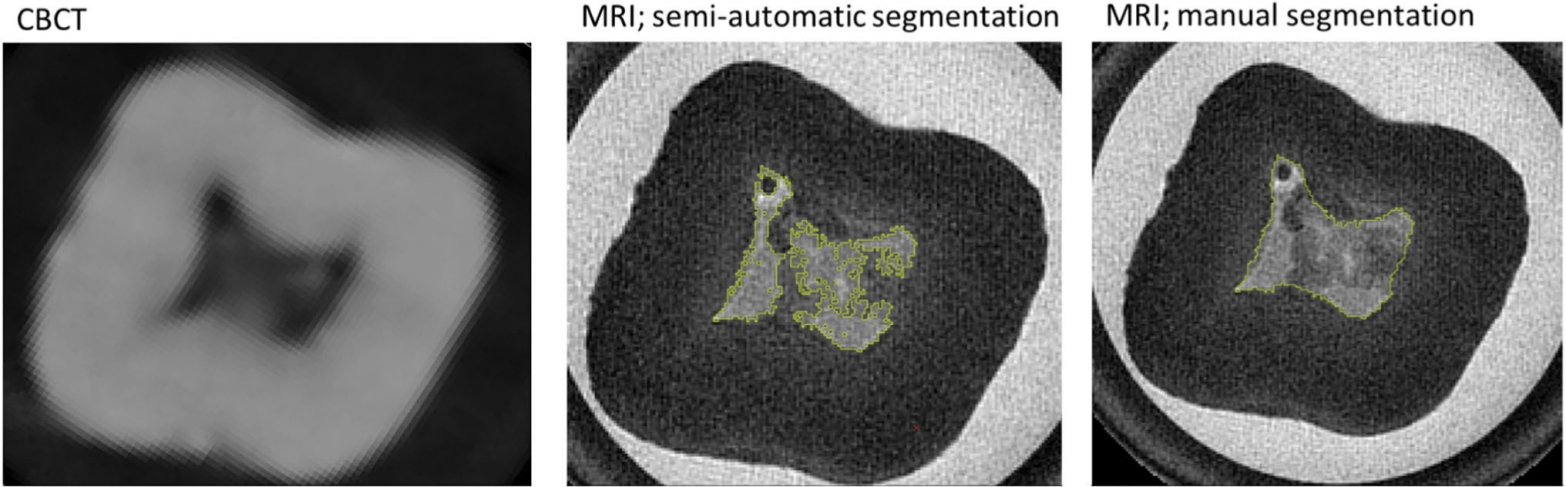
Tooth 37. Tooth of 54-year-old male; transversal view. (“smoothing”; none). Left: CBCT-method. Middle: MRI-method, semi-automatic-setting (yellow line). Right: MRI-method, manual-setting (yellow line). The higher information content about the interior of the dental pulp in the MRI, represented by substantial differences in intensity, leads to a representation of the pulp in the semi-automatic segmentation that cannot be reconciled with the anatomical conditions
